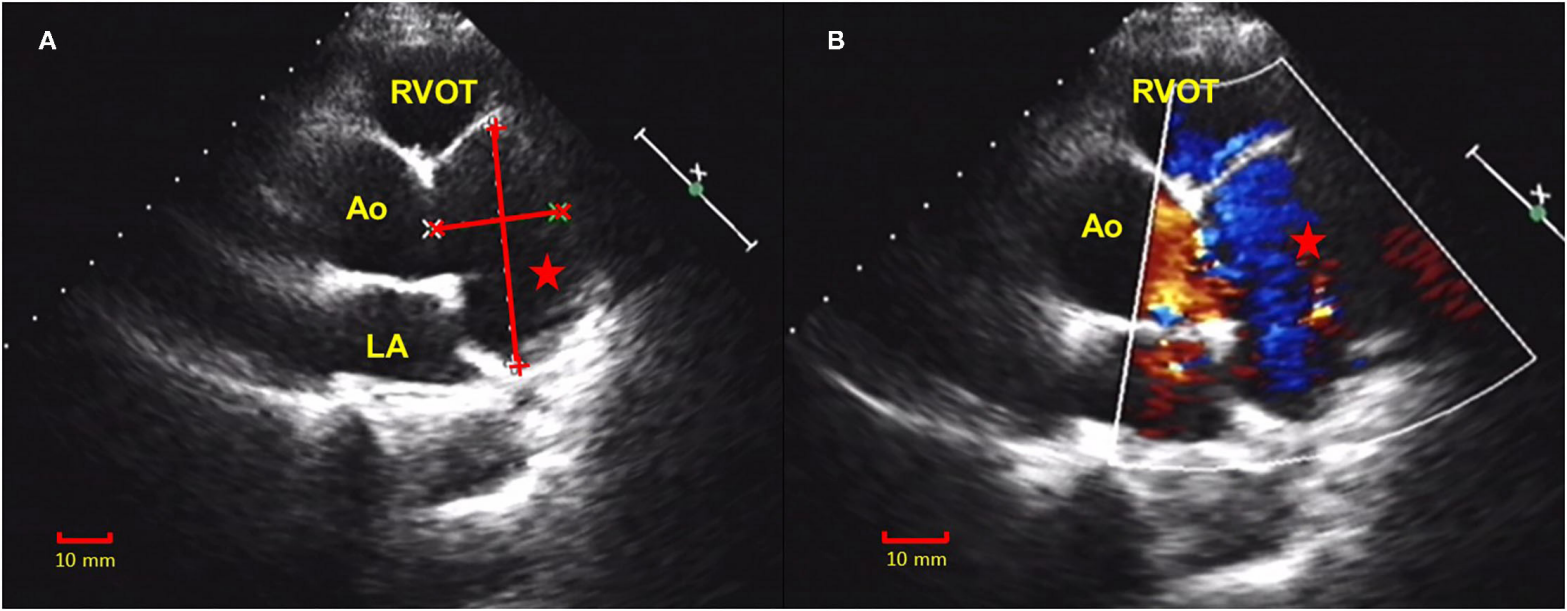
Transthoracic echocardiogram (TTE) showed a giant aneurysm at the parasternal aortic short axis view that was originated from the left sinus of Valsalva (A). Communication of the blood flow was detected between the aneurysm and the aorta (B). TTE, transthoracic echocardiogram; RVOT, right ventricle outflow tract; Ao, aorta; LA, left atrium; red star, sinus of Valsalva aneurysm (SoVA).
